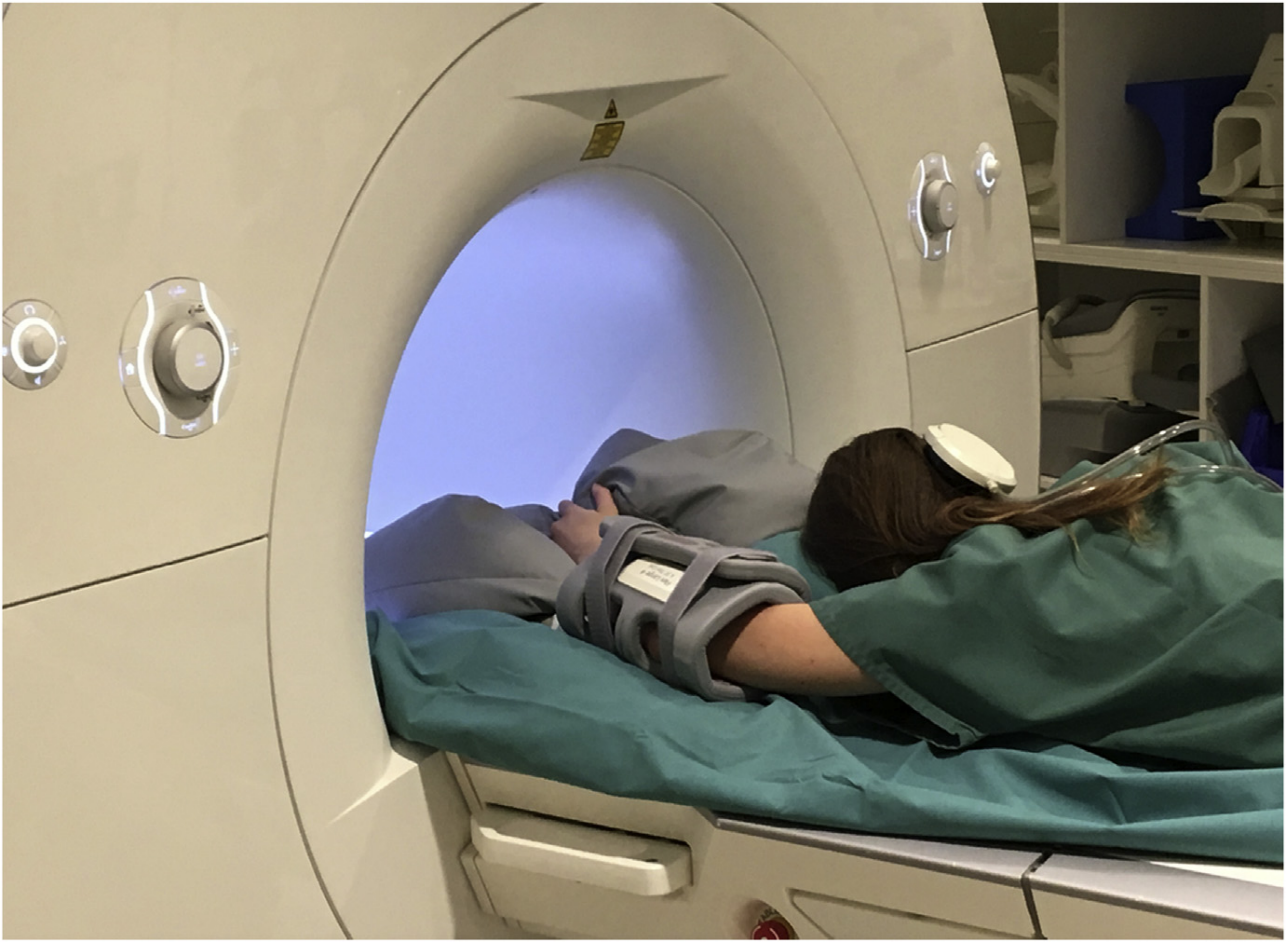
MR scanning positioning: The figure shows a volunteer, who has been positioned in prone position for MR imaging of the ulnar nerve at the level of the elbow - the so called “superman position” - with the arm extended overhead. A 4-channel flexible coil (Siemens Healthcare) is placed around the elbow.
